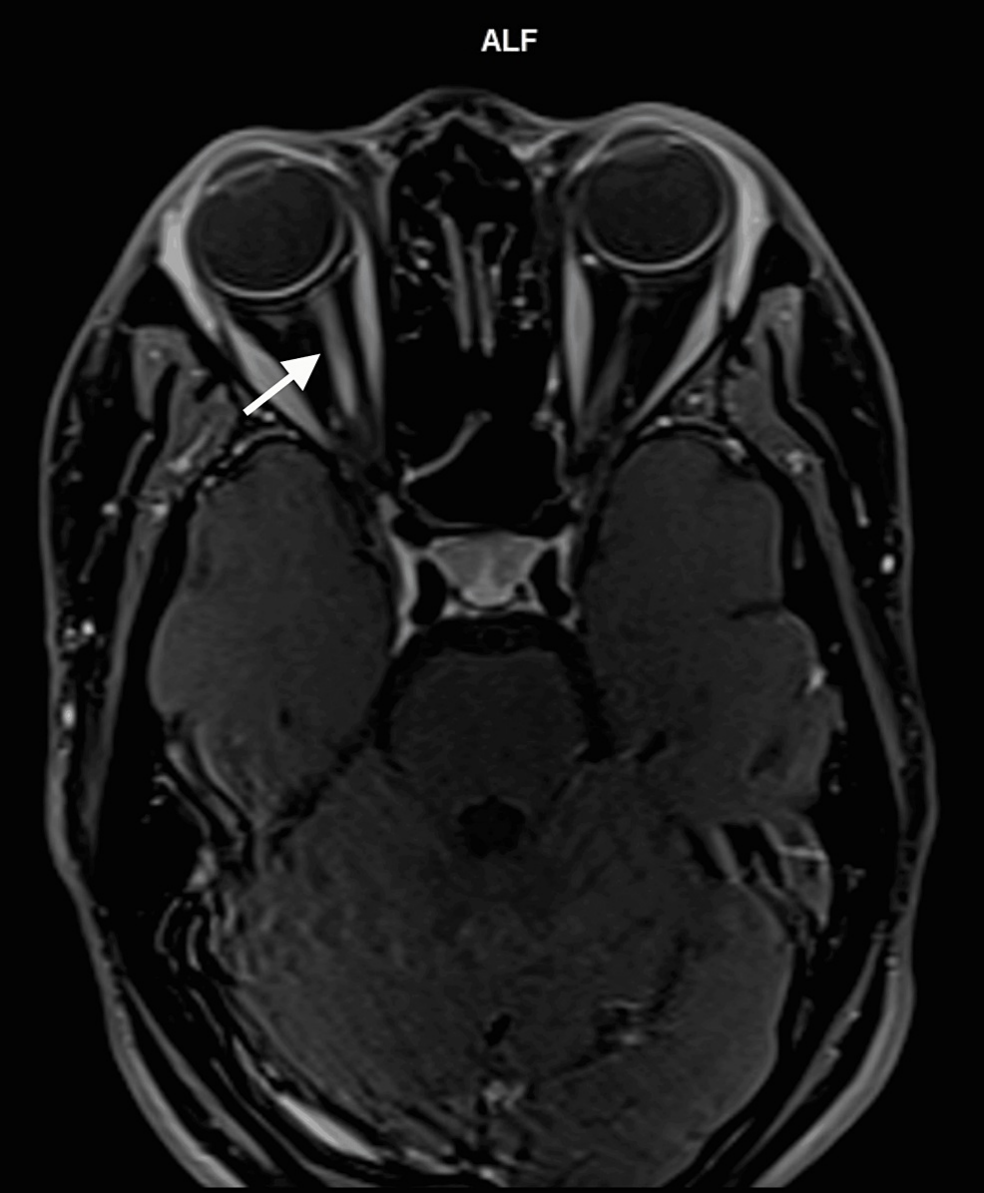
MRI film axial view: right optic nerve enhancement The white arrow shows the hyperintense right intraorbital segment of the right optic nerve.
